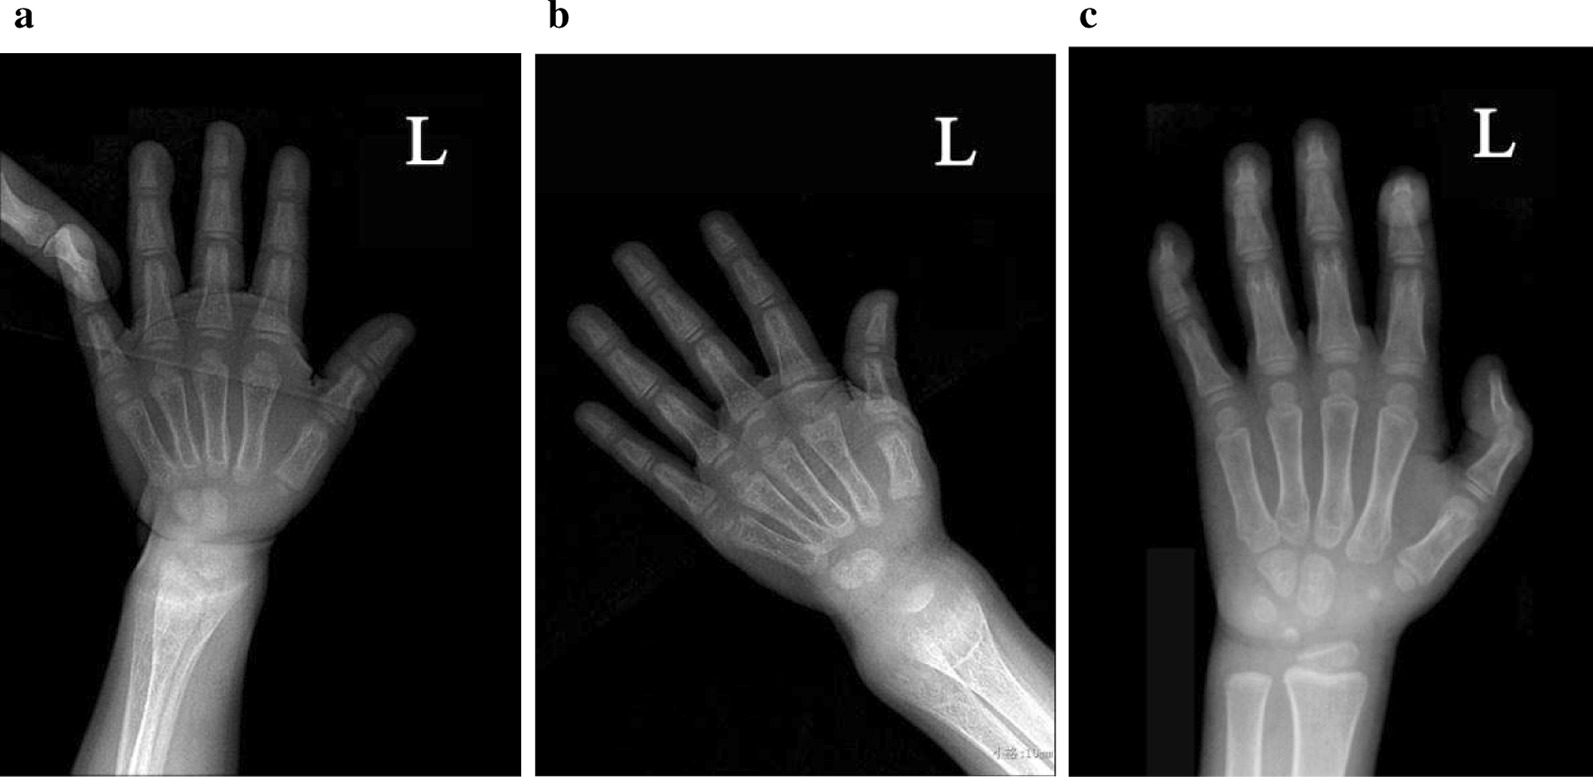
Radiographs of the patient 1 with VDDR-IA. a Before treatment, wrist radiograph disclosed the vanishing of the zone of provisional calcification in distal metaphysic, rarefaction of bone trabecula and thinness of the cortical bone in the ulna and radius. b After 3 months of treatment, improvement of bone trabecula is obvious. c After 1 year of treatment, wrist radiograph showed reoccurrence of the zone of provisional calcification in distal metaphysis, and improvement of bone trabecula and thickening of the cortical
